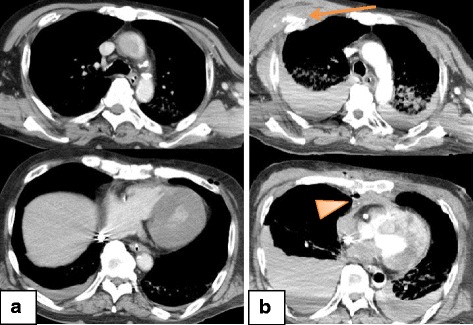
Computed tomography findings from Patient 1. a Contrast-enhanced computed tomography after the extracorporeal cardiopulmonary resuscitation revealed bilateral multiple rib fractures, a sternum fracture, and right pleural effusion without contrast extravasation. b Repeat contrast-enhanced computed tomography after coronary angiography revealed contrast extravasation at the anterior chest wall (*arrow*) and mediastinum (*arrowhead*), with increases in the size of these hematomas
